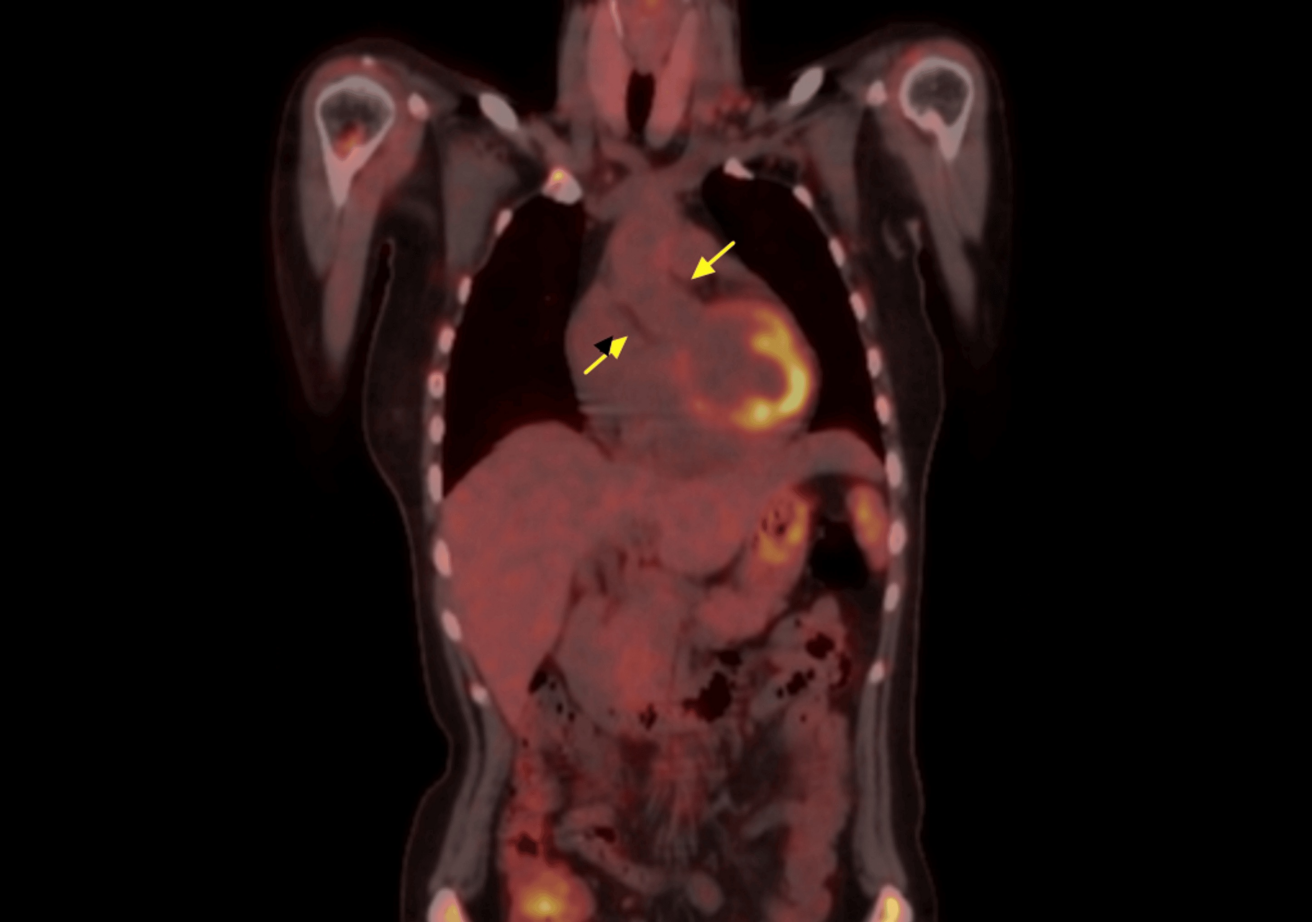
Coronal view of FDG-PET/CT No FDG uptake was observed in the wall of the ascending aorta or in any other arteries on PET/CT (arrow). FDG: fluorodeoxyglucose, PET/CT: positron emission tomography/computed tomography
